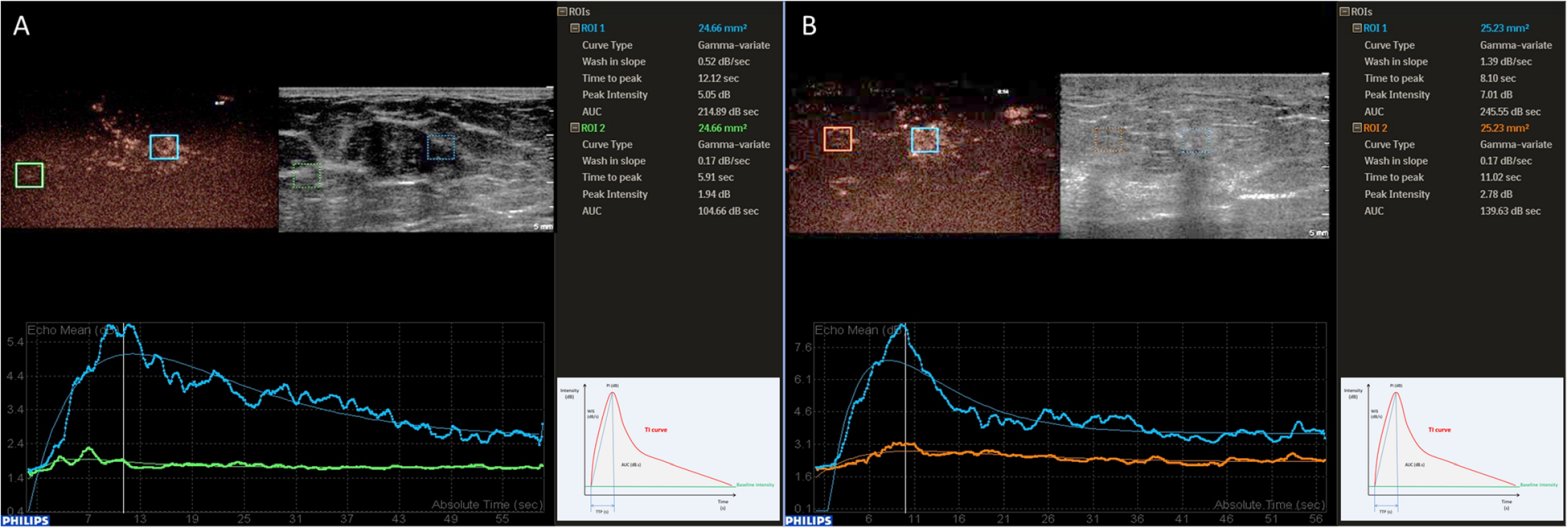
Examples of quantitative data acquisition using ROI. Fibroadenoma in a 39-year-old female (a). Strikingly rich vascularization of the lesion (blue ROI) compared to minimal blood perfusion in surrounding breast tissue (green ROI). Gradual enhancement and a gradual wash out of contrast agent (corresponding TI curves below). Invasive carcinoma of no special type (NST) in a 59-year-old female (b) with Pronounced enhancement of the lesion and adjacent tissue (blue ROI), the lesion is ill-defined. After fast enhancement of the tumor, early wash-out can be observed (corresponding TI curve below). The enhancement of surrounding breast tissue is (orange ROI, corresponding TI curve below). Abbreviations: ROI, region of interest; TI, time intensity curves; NST, invasive carcinoma of no special type; CEUS, contrast-enhanced ultrasound
